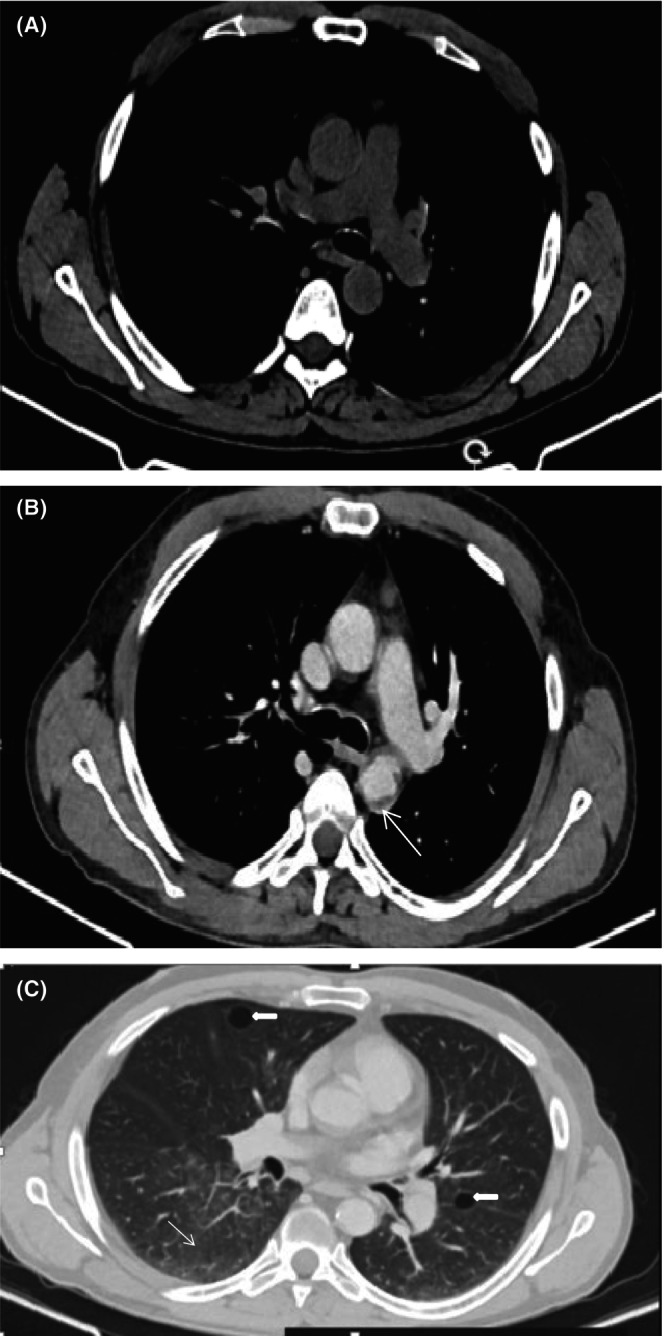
Axial pre‐contrast (A) and post‐contrast (B) chest CT shows soft‐tissue encasement of the aortic arch and descending thoracic aorta (coated aorta sign) (arrow). On lung window (C), there are randomly distributed multiple different sized lung cysts (thick arrow) with right lower lung ground glass opacities (thin arrow).
